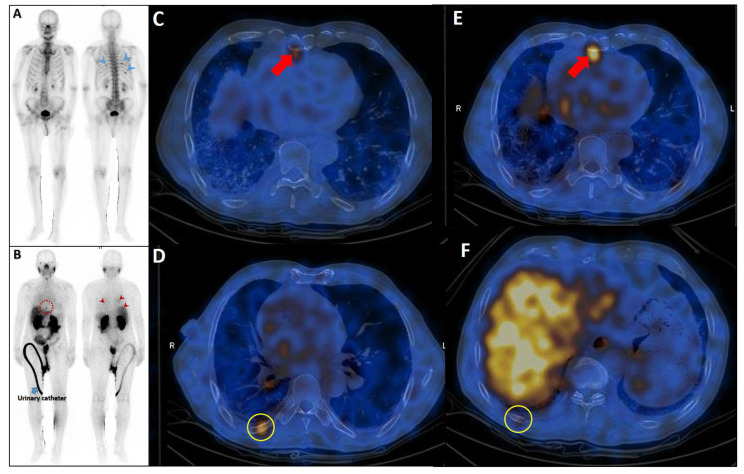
Complementary role of PSMA SPECT/CT to bone scan in a patient with equivocal rib metastases. A very-high-risk prostate cancer patient (Gleason score: 5 + 4, serum PSA = 43 ng/dL) was referred for staging. The bone scan revealed at least three foci of osteoblastic activity in the ribs posteriorly ((A), blue arrowheads). Subsequently, the patient underwent PSMA SPECT/CT (B–F), confirming a mild PSMA avidity in the mentioned regions ((B), red arrowheads) with subtle sclerotic changes in two of them ((D,F), yellow circles). Moreover, another focus of uptake was noticed in the xiphoid process ((B), dashed red circle; (C,E), red arrows). Given the patient’s clinical history, presence of lymph nodes metastases (not shown), and possibility of xiphoid involvement, the patient was finally concluded as having metastatic rib lesions in the bones.
